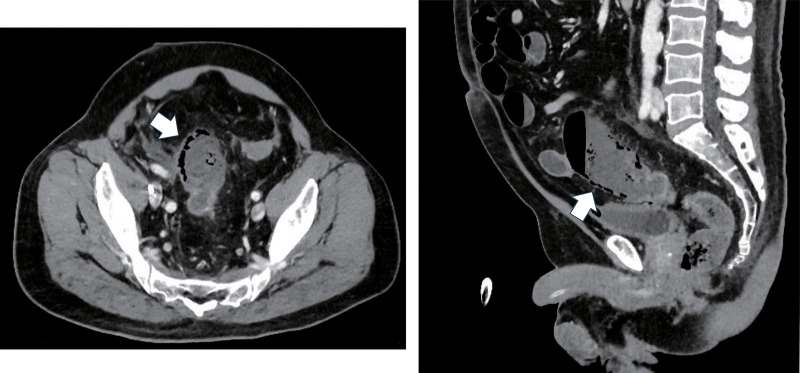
Computed tomography (CT) imaging revealed pneumatosis intestinalis of the sigmoid colon (white arrow). This CT scan was performed on the second day after the dive when symptoms had worsened.
